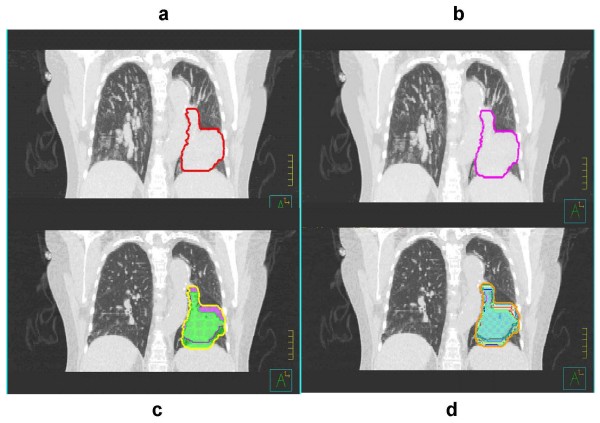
Delineation of IGTV for stage III lung tumors based on (a) IGTV_MIP_, (b) IGTV_MIP-Modified_, (c) IGTV_2Phases_, and (d) IGTV_AllPhases _of a 4-D CT data set. MIP-based contours, as shown in panels (a) and (b), are as they appear on the MIP data set. Phase-based contours, as shown in panels (c) and (d), are registered to the peak exhalation phase of the 4D-CT data set.
